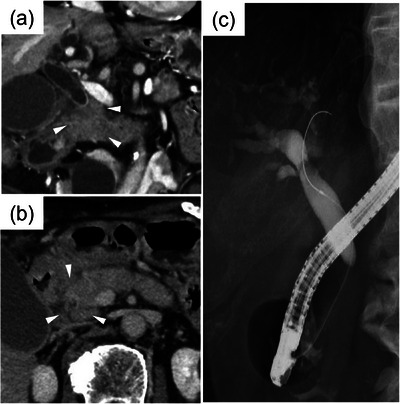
Computed tomography and endoscopic retrograde cholangiopancreatographyimages. Enhanced computed tomography showed stenosis in the common bile duct (arrowhead) by a poorly marginated mass around the pancreatic head (approximately 2 cm wide and 3 cm long), which also involved the second part of the duodenum (a, b). Endoscopic retrograde cholangiopancreatography showed a 5‐cm distal common bile duct stricture and dilated common and intrahepatic bile ducts (c).
